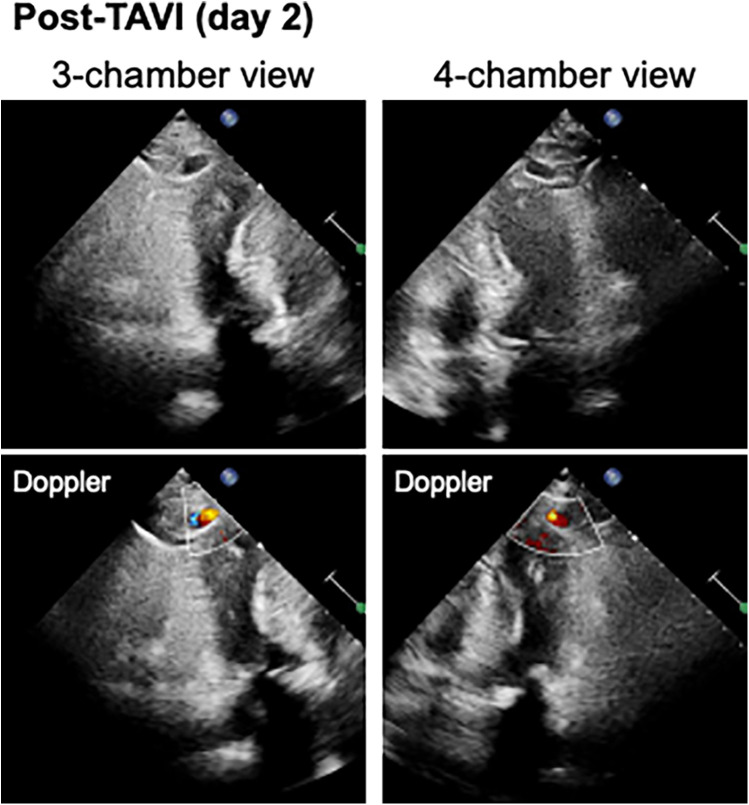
The color doppler echocardiography visualized a cavity and abnormal blood flow inflow within the apical structures consistent with a pseudoaneurysm.
